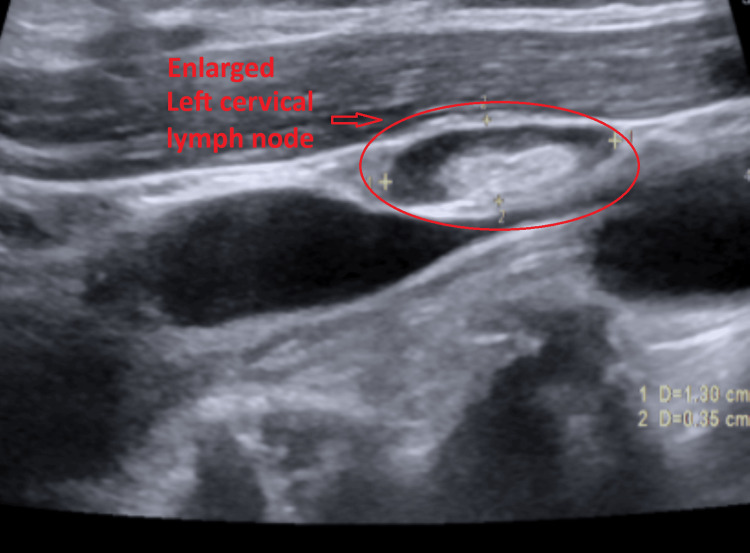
Ultrasound image of the largest left cervical lymph node
